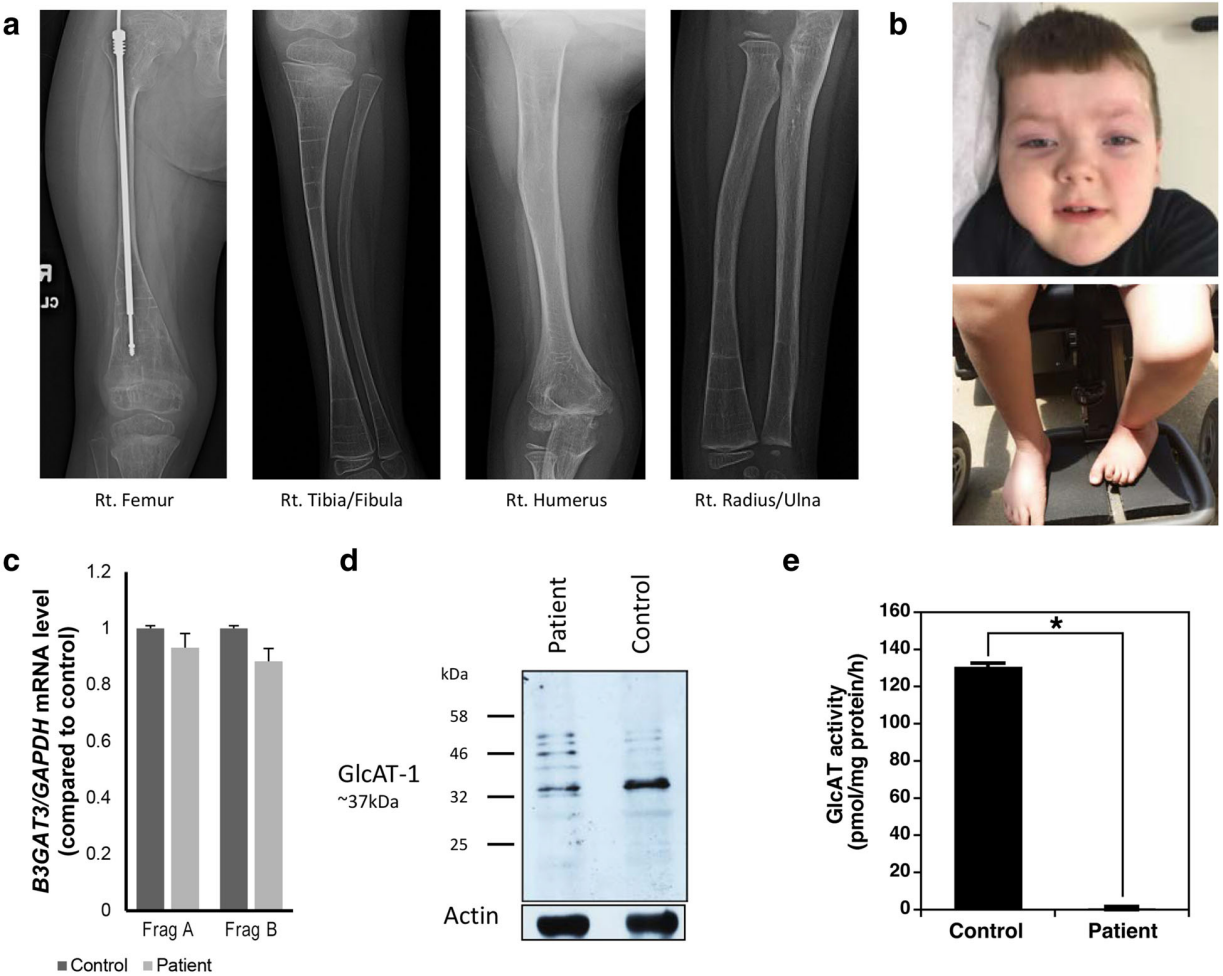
a X-rays revealed severely diffusely demineralized bones, along with multiple growth arrests lines. Stabilization rods are in both femurs and bilateral bowing of the tibiae and fibulae are evident (*right pictured*). b Cmh000720 is mildly dysmorphic with downslanting palpebral fissures (*left*), short neck, and curved lower extremities. While able to ambulate with a walker and physical assistance, he is wheelchair-bound. c mRNA expression of B3GAT3 in primary fibroblasts in cmh000720 was not statistically different from control using two sets of primers, whereas western blotting (d) showed a decrease in overall protein. e Glucuronyltransferase activity was determined by incorporation of [^14^C]GlcA into the Galβ1-3Galβ1-*O*-methyl. The control sample utilized an enzyme source from the fibroblasts from a healthy control subject. P* < 0.0001
